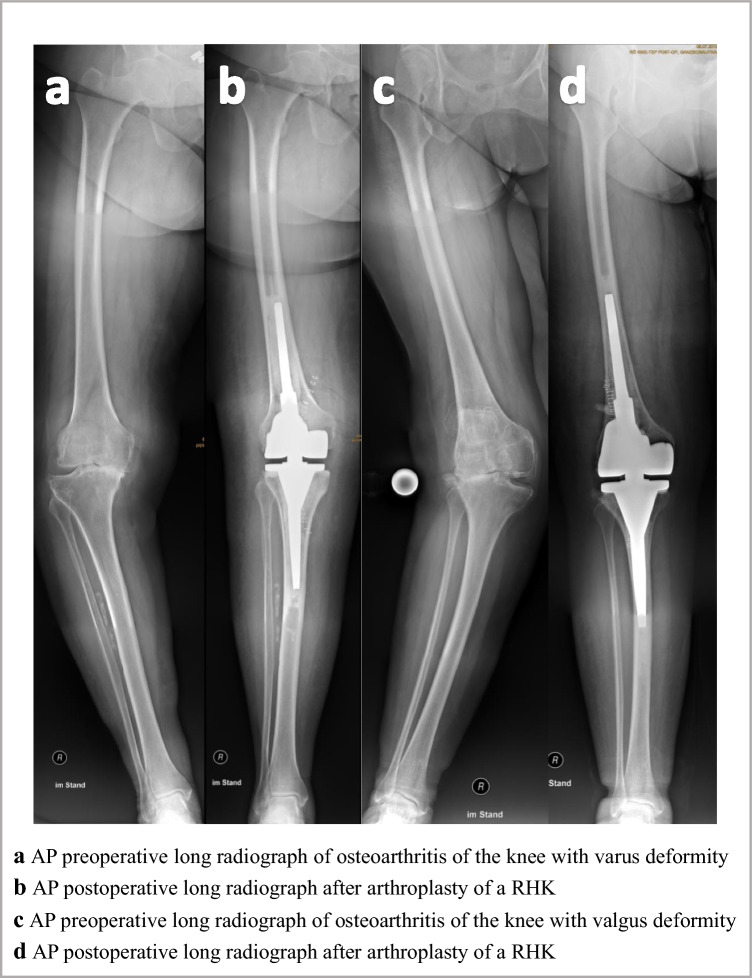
Images a AP preoperative long radiograph of osteoarthritis of the knee with varus deformity.  b AP postoperative long radiograph after arthroplasty of a RHK. c AP preoperative long radiograph of osteoarthritis of the knee with valgus deformity. d AP postoperative long radiograph after arthroplasty of a RHK
